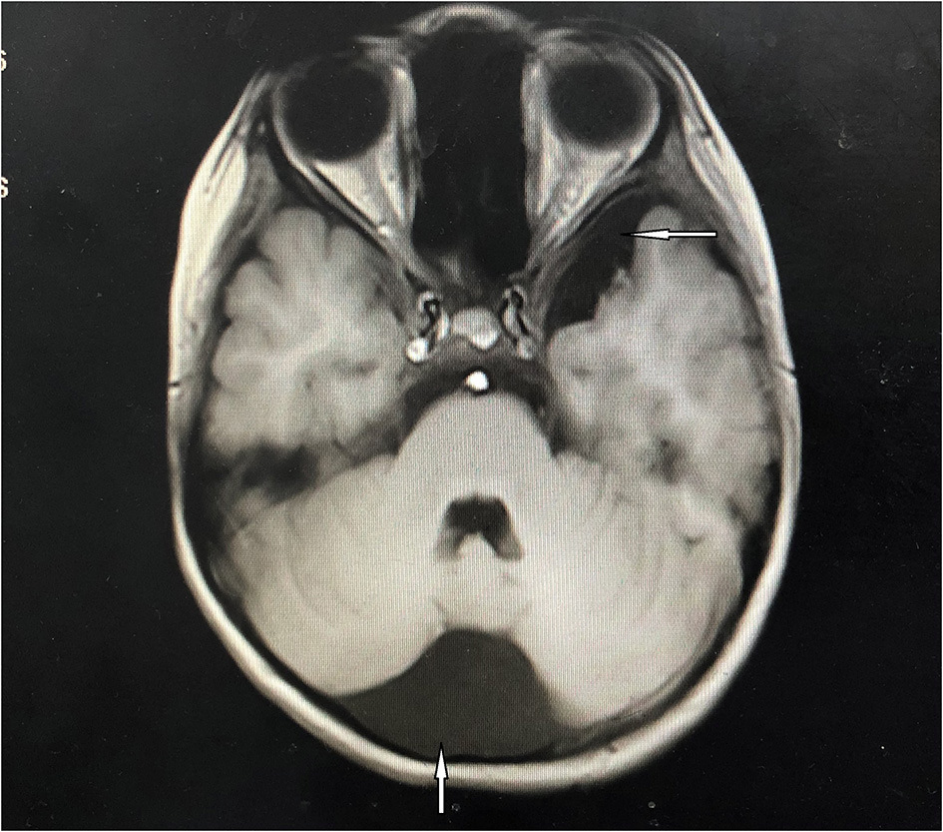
Cranial magnetic resonance imaging. Arachnoid cysts of occipital (the arrow underlying) and left middle cranial fossa (the arrow above) had been detected.
